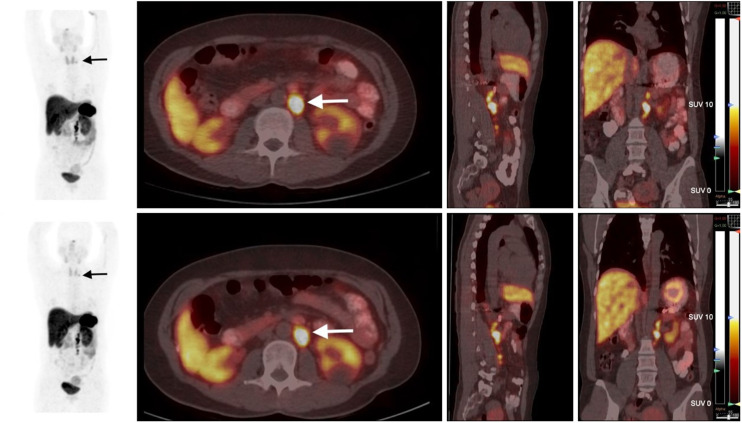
Example of 2 examinations, with cyclotron-produced (top row) and generator-produced (bottom row) ^68^Ga-DOTATATE PET/CT. From left to right in each row maximum-intensity projection (MIP), axial, sagittal and coronal planes. Examinations were performed 16 mo apart on same patient, who was being followed after removal of left pheochromocytoma but had secondary supraclavicular (black arrows) and retroperitoneal (white arrows) node metastasis.
